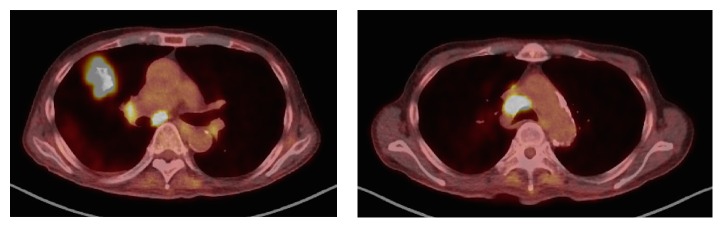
Lung metastasis and mediastinum lymph node metastases are depicted in the PET-CT.
